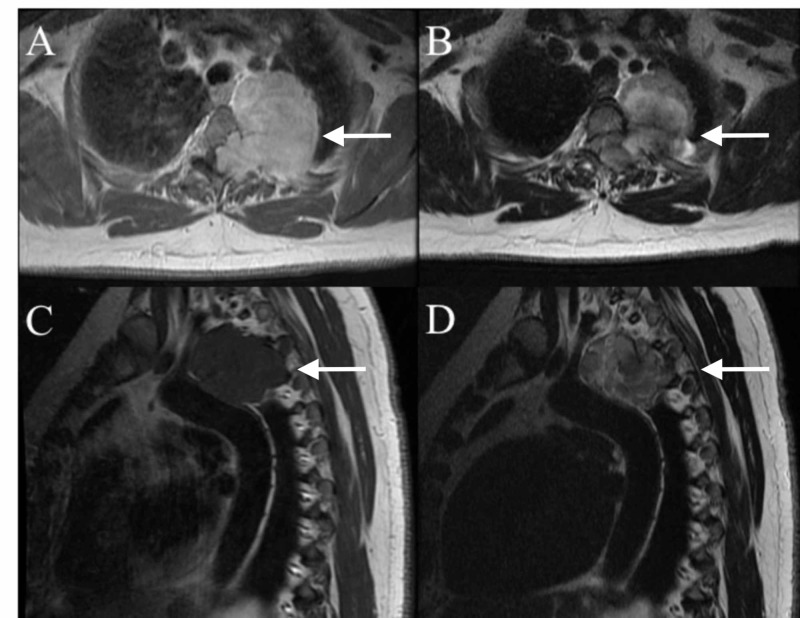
Preoperative MRI thoracic spine with and without contrast Preoperative T1 axial MRI with gadolinium (A) and T2 axial MRI without contrast (B) showing dumbbell schwannoma intraspinal and extraspinal components. Preoperative T1 (C) and T2 (D) sagittal MRI showing dumbbell schwannoma extending into the posterior mediastinum adhering to the thoracic aorta. MRI, magnetic resonance imaging
